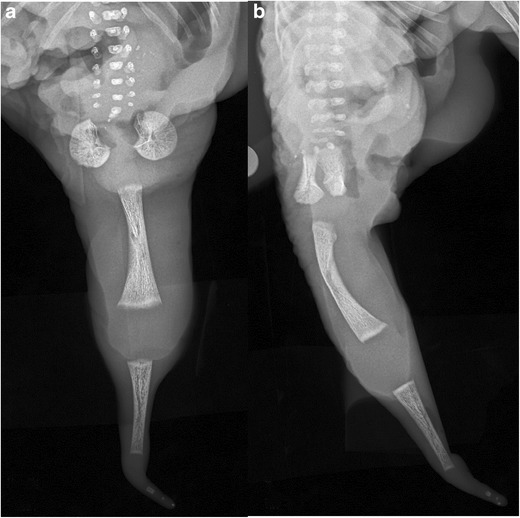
a-b: Sirenomelia (“mermaid sequence”): Post-mortem AP (*a*) and lateral (*b*) radiographs of the lower body of a prematurely terminated foetus demonstrates absent sacrum and fused bilateral lower extremities. This anomaly is incompatible with life
